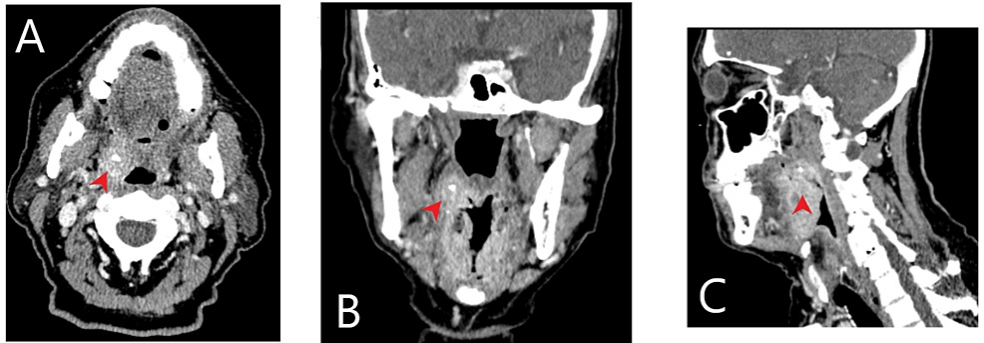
CT of the soft tissue neck with contrast showing peritonsillar fluid collections on the right side. Red arrowhead: peritonsillar fluid collection. A) Axial. B) Coronal. C) Sagittal
